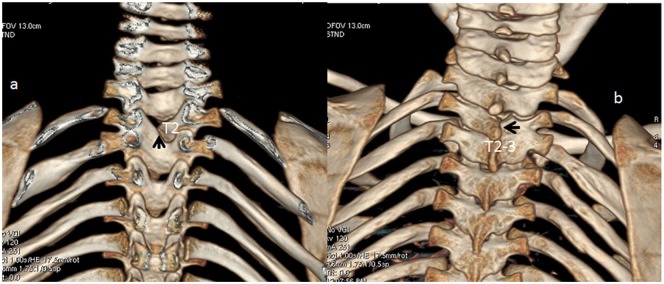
Thoracic kyphosis. Three-dimensional (3D) reconstruction computed tomography (CT) scan in a 6-year-old boy with autosomal dominant Robinow syndrome who presented with thoracic kyphosis showed malsegmentation of T2/3 (anterior view-arrow). The 3D reconstruction CT scan (posterior view) confirmed the malsegmentation along the T2/3 and not associated with rib fusions.
